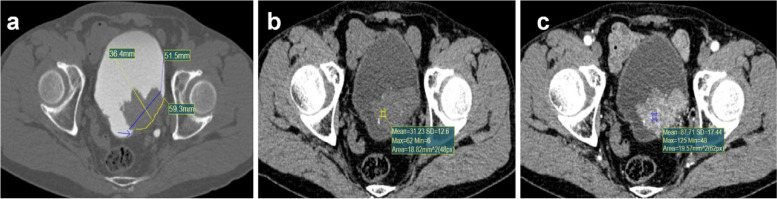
A 66-year-old male patient who underwent TURBT and was confirmed as Bladder urothelial carcinoma. a Axial excretory phase image showed the lesion was located at the left posterior wall, the junction between lesion edge and bladder wall was unsmooth (arrow), the maximum transverse diameter of this lesion was 5.15cm, the maximum longitudinal diameter was 3.64cm (Transverse diameter/longitudinal diameter=1.41) and the tumor-wall interface was 5.93cm (yellow curve). b The non-enhanced CT value of this lesion was 31.23HU. c The arterial phase CT value of this lesion was 87.71HU, the arterial reinforcement rate was 1.81 ([87.71-31.23]/31.23)
